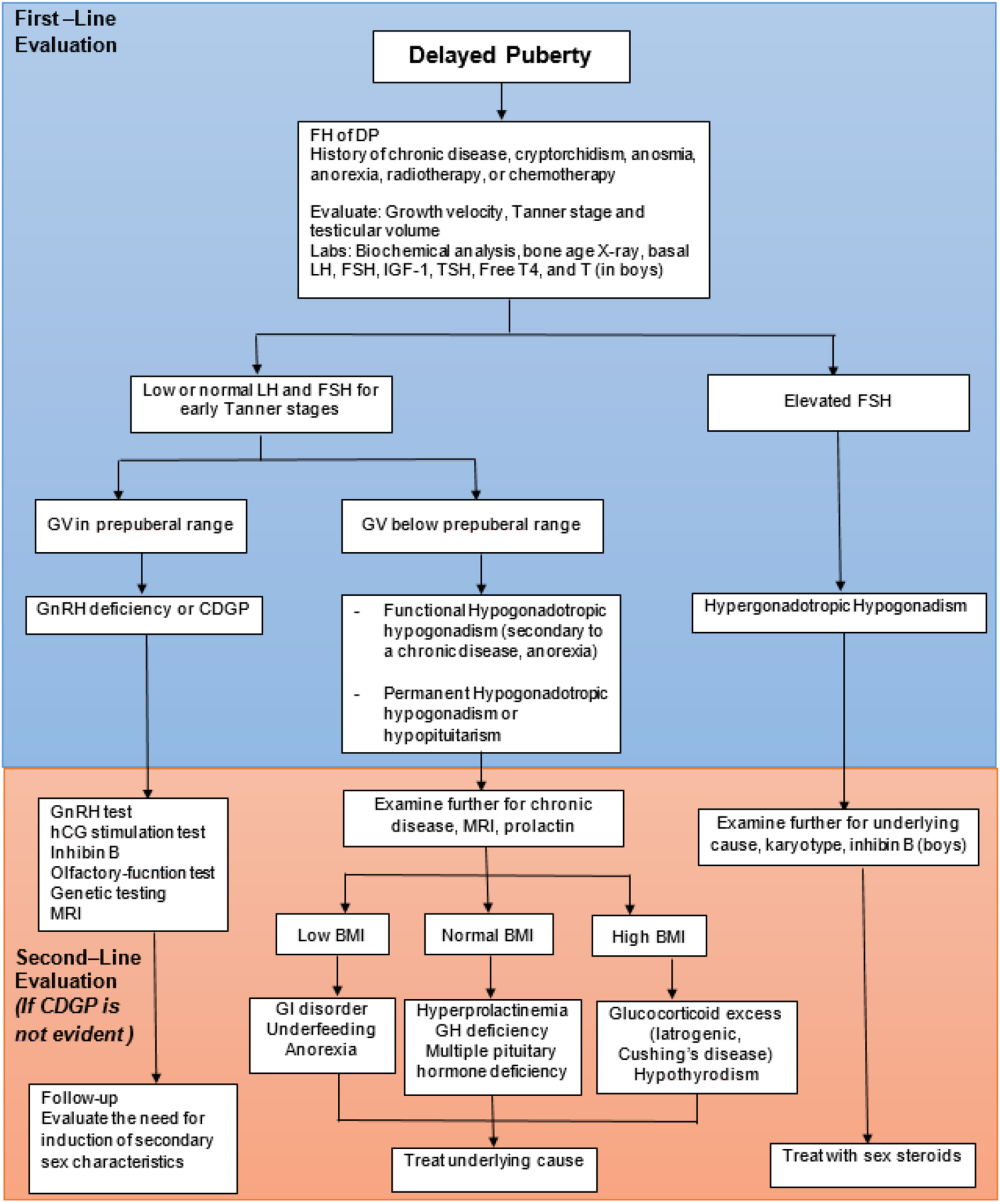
Algorithm for the evaluation of a patient with delayed puberty. BMI, body mass index; CDGP, constitutional delay of growth and puberty; FSH, follicle-stimulating hormone; GH, growth hormone; GI, gastrointestinal; GnRH, gonadotropin-releasing hormone; hCG, human chorionic gonadotropin; IGF-1, insulin-like growth factor 1; LH, luteinizing hormone; MRI, magnetic resonance imaging (3). From *New England Journal of Medicine*, Palmert MR & Dunkel L, ‘Delayed puberty’, Volume 366, Pages 443–453. Copyright © (2012) Massachusetts Medical Society. Reprinted with permission from Massachusetts Medical Society.
